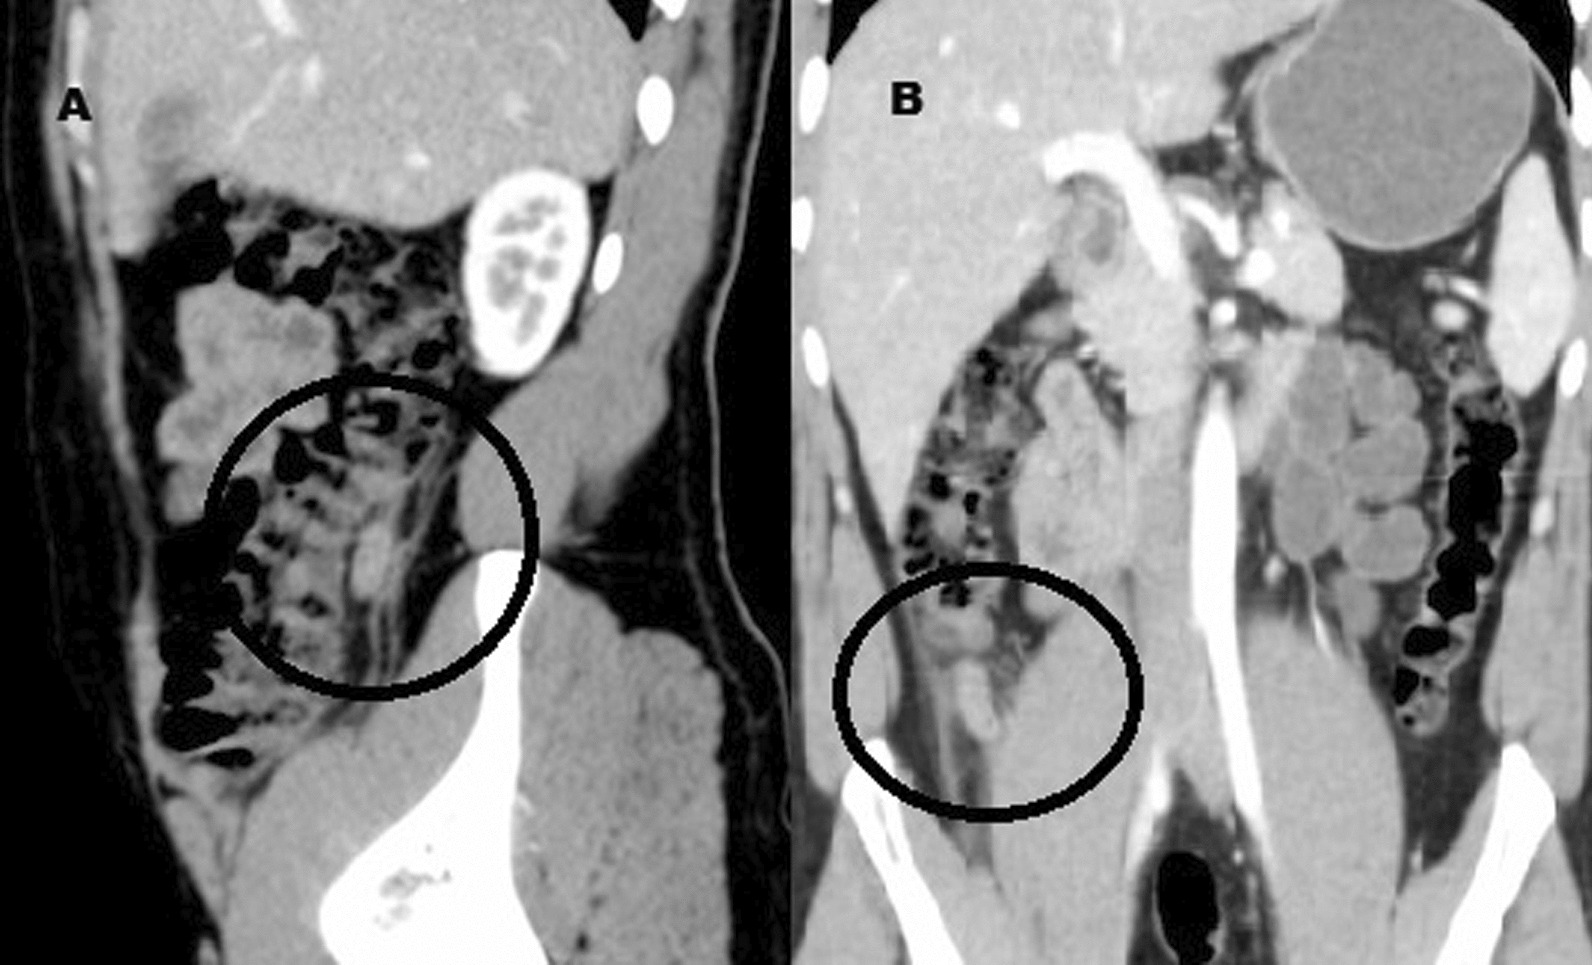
CT abdomen showing inflamed blind ended loop (circle) emerging from the posterior aspect of the middle part of the right colon (sagittal section; A) at the right lumber region (at a distance measuring about 3.5 cm distal to the inferior surface of the liver and about 7 cm proximal to the ileo-cecal junction, coronal section; B) measuring about 1 cm at maximum diameter and about 5 cm in length
